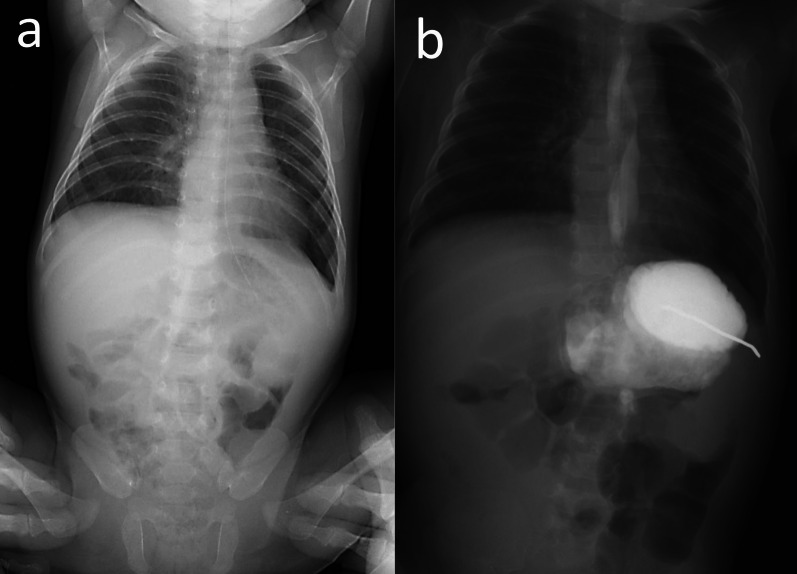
Postoperative X-ray (a) and upper gastrointestinal series (b). a Postoperatively, the stomach was correctly positioned without volvulus. b Gastroesophageal reflux persisted for a while
